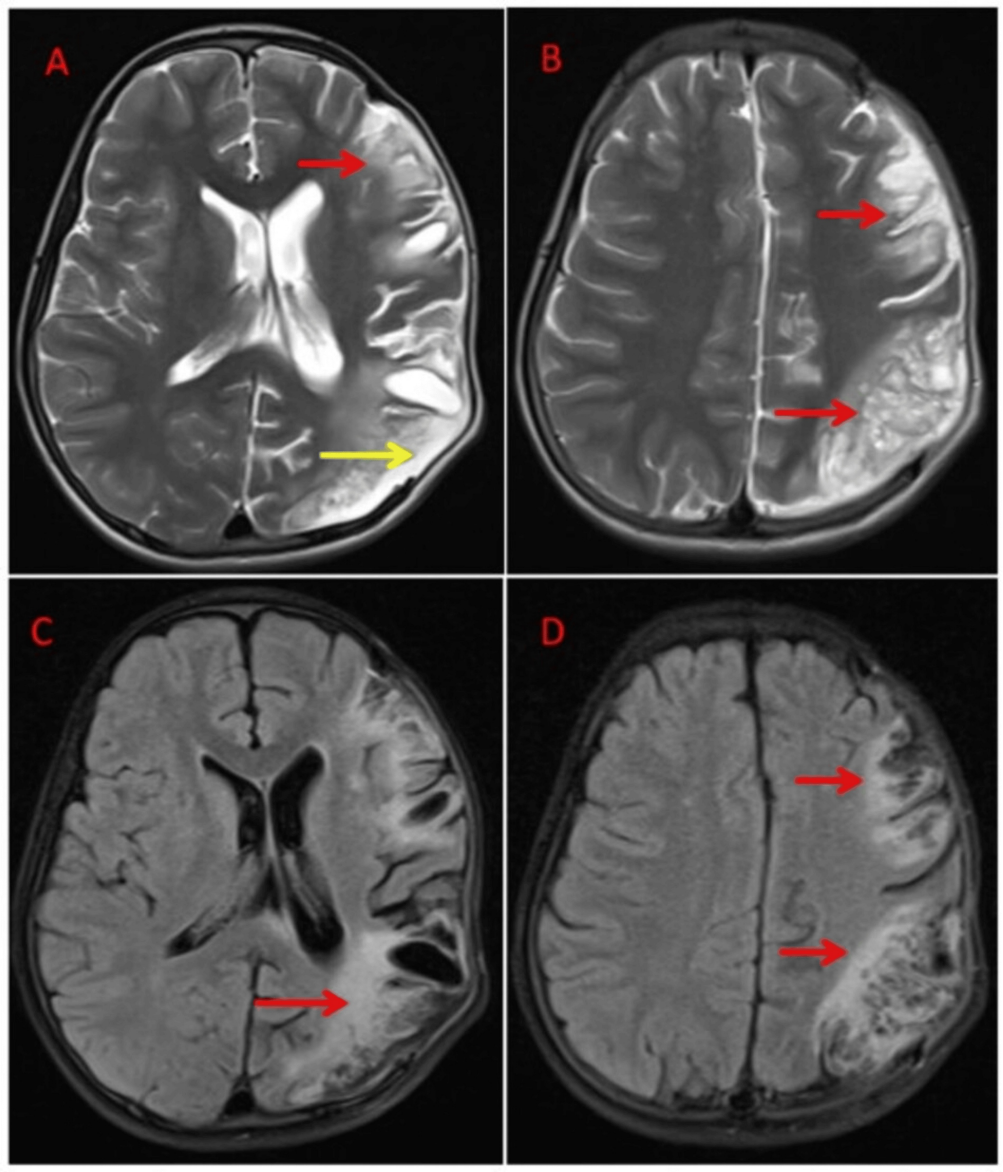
(A-B) Axial T2, (C-D) Axial FLAIR showing extensive areas of encephalomalacia following CSF intensity on T2/FLAIR sequences, with surrounding gliosis appearing hyperintense on T2/FLAIR in the left fronto-parietal region (marked by red arrows). A large craniectomy defect in the left fronto-parietal region with mild herniation of parenchyma (marked by yellow arrow). FLAIR: fluid-attenuated inversion recovery, CSF: cerebrospinal fluid.
